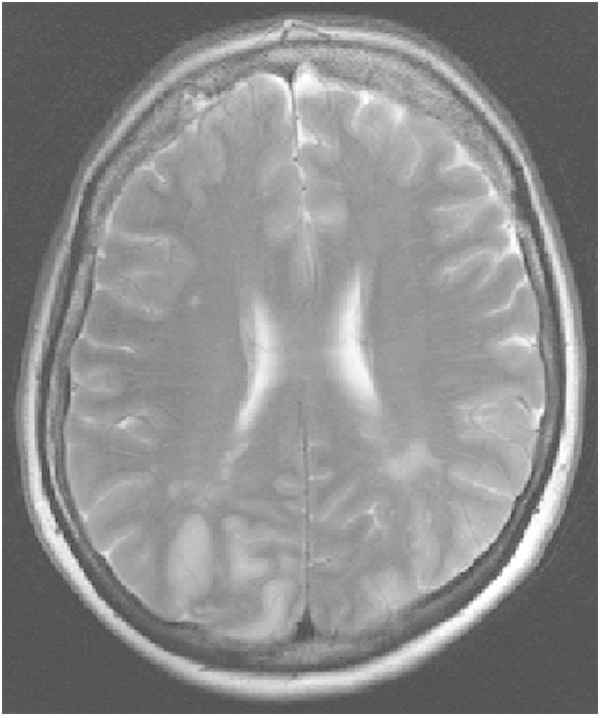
Primary MRI, T2-weighted image.
